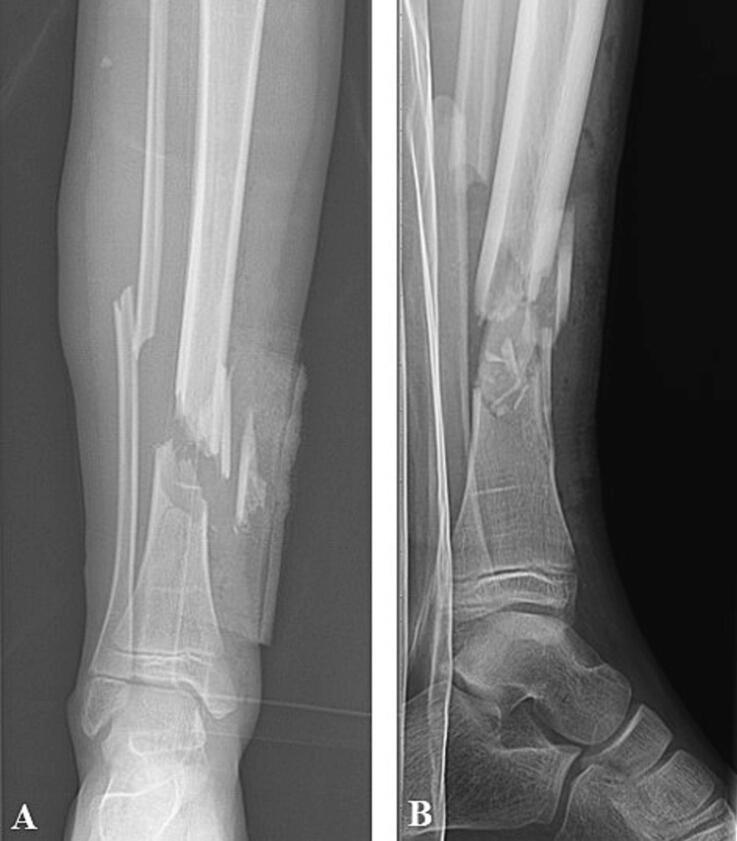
Trauma X-ray, anterior-posterior (A) and lateral (B) views, showing a right comminuted tibia fracture including a ± 5.0 cm tibial TBD and a dorsal-lateral translated distal fibula fracture.
